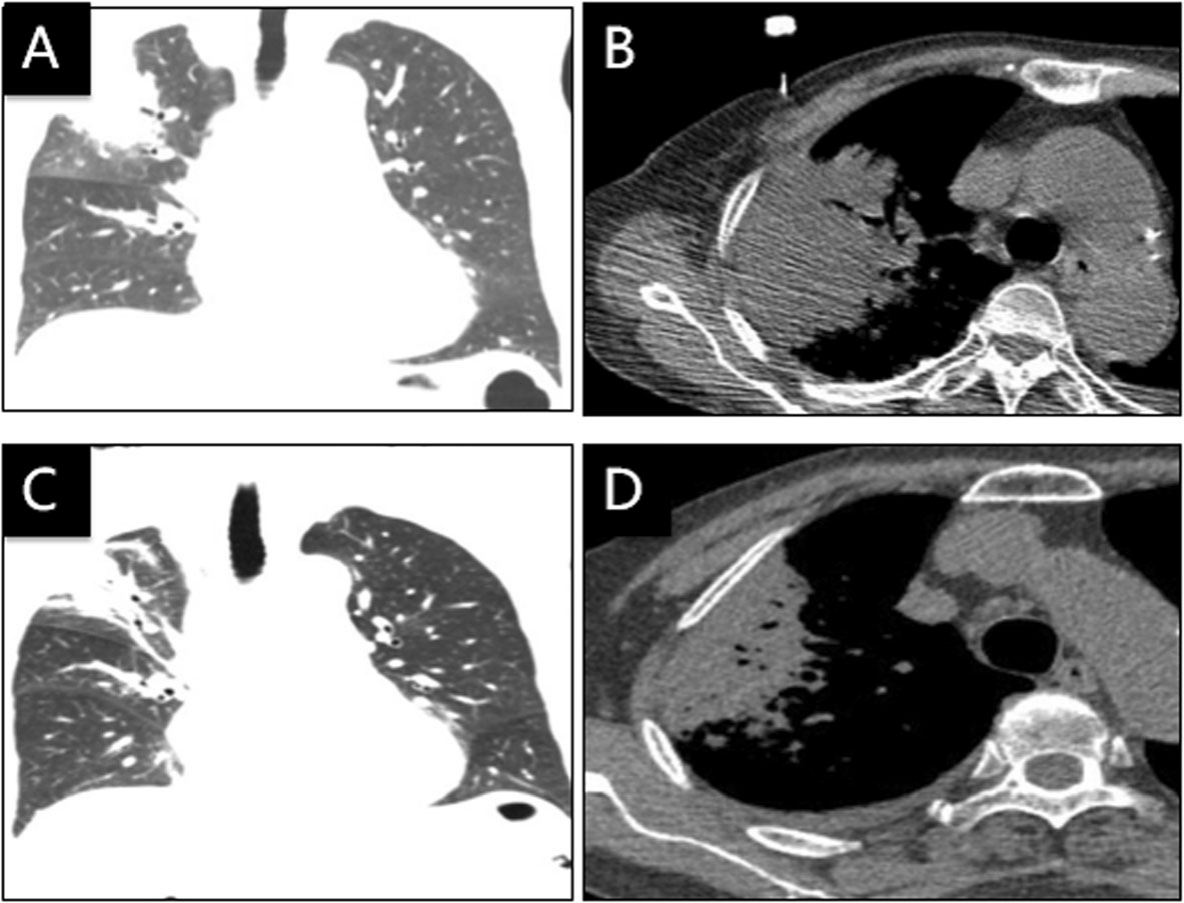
Chest CT of case 3: a, b On the day of admission, c, d 9 days after treatment with doxycycline plus moxifloxacin
